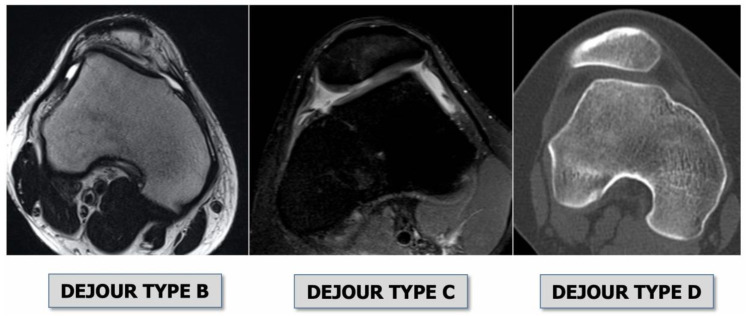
Types trochlear dysplasia on MRI axial view, as classified by Dejour [7].
